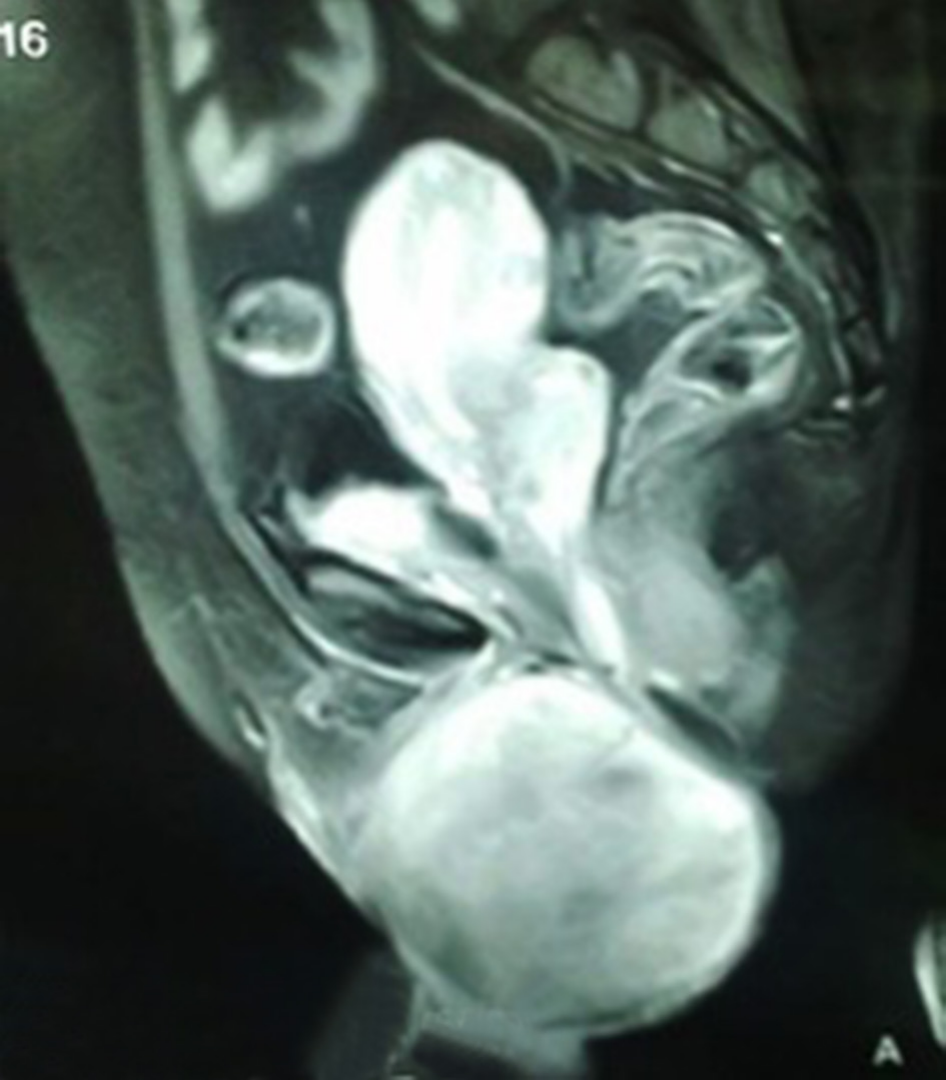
Magnetic Resonance Imaging (T2-Weighted, Sagittal Fat Suppression Image) Showing a Larger Mass Arising from Clitoris With Compression of the Urethra
